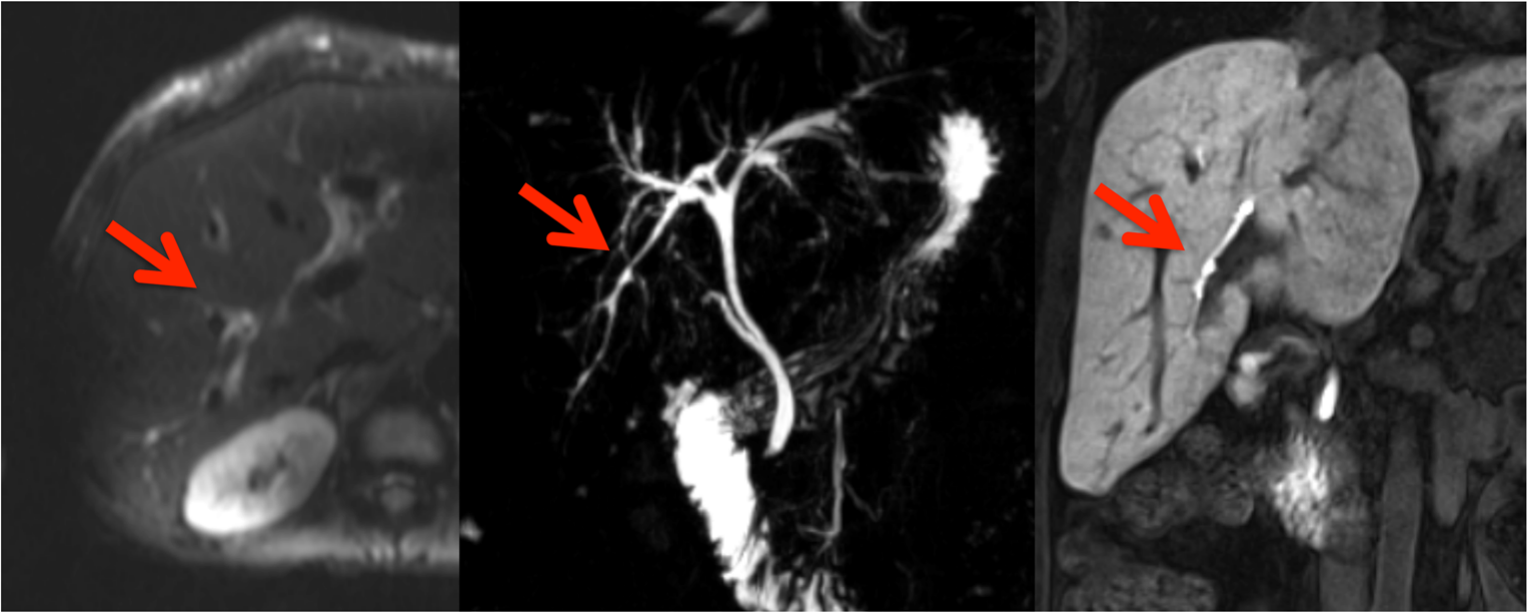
In this 25-year-old male cystic fibrosis (CF) patient periportal tracking (PPT) is shown on the diffusion-weighted images, demonstrating a band-like hyperintense signal alteration along the portal triad. Bile duct abnormalities (BDA) are shown in the T2-weighted magnetic resonance cholangiopancreaticography images and in T1-weighted images, in the hepatobiliary phase (20 min after the administration of gadoxetic acid) by contour irregularities of the intrahepatic bile ducts
